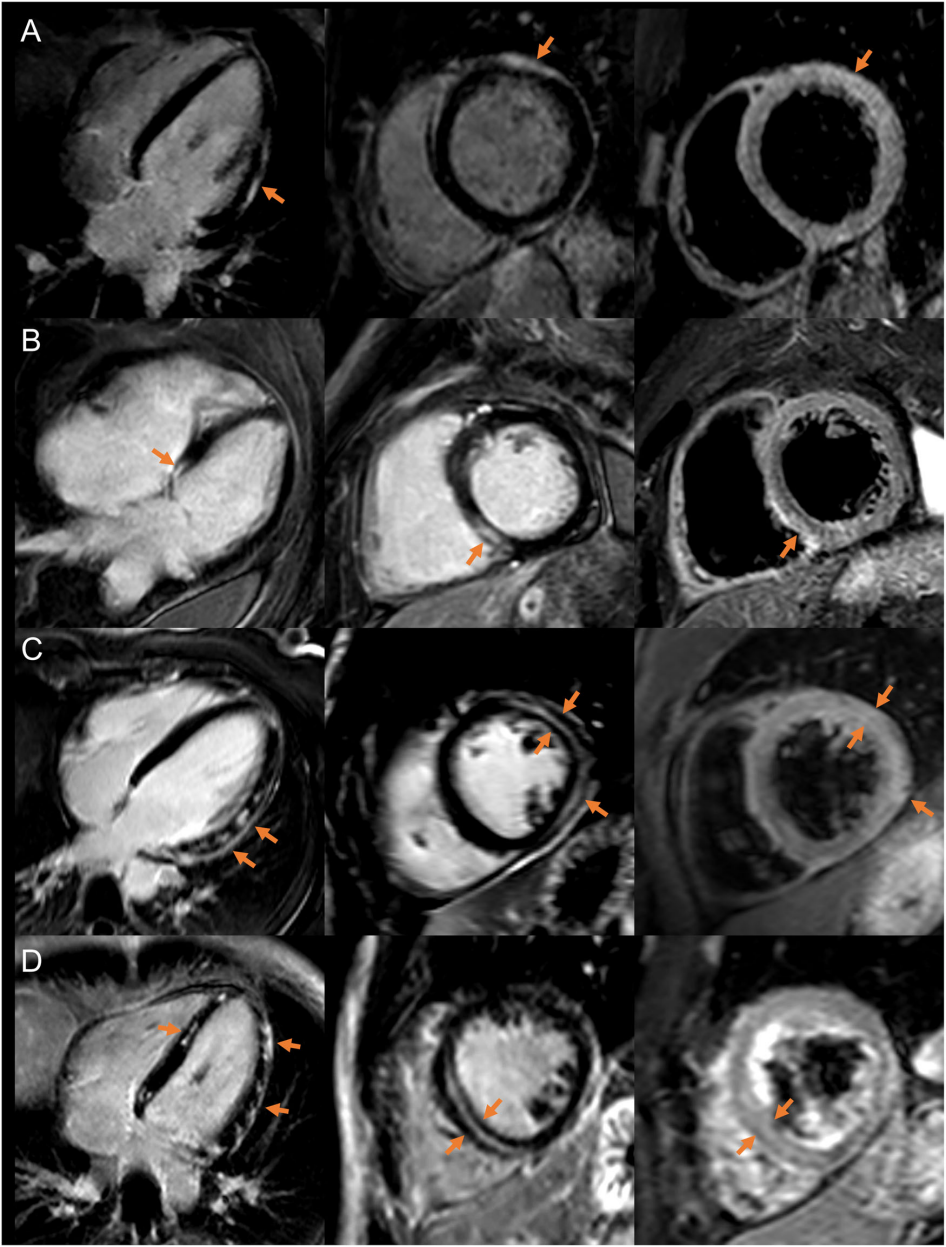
Cardiac magnetic resonance imaging examples of patients with different LGE patterns: (A) subepicardial, (B, D) midwall with septal involvement, and (C) subepicardial and midwall. Corresponding LGE lesions with focal edema are marked with orange arrows, respectively. Left column: LGE in four-chamber view; middle column: LGE in short axis view; and right column: fat-suppressed T2 sequence in short axis view
